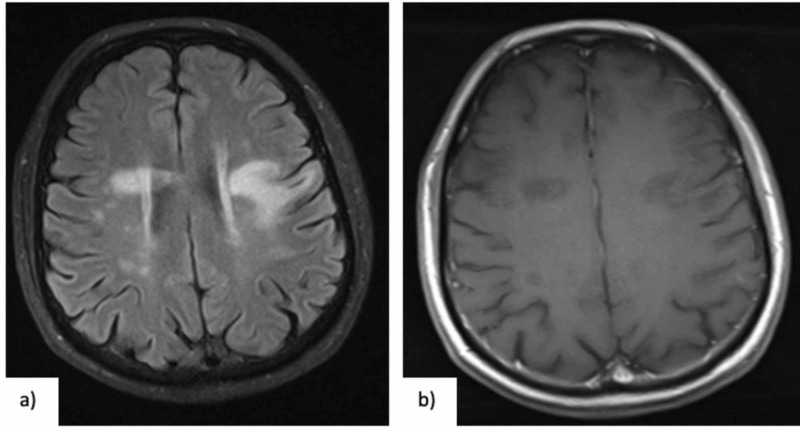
(a) Brain MRI (05-07-2017) performed after corticotherapy, showing reduction of previous lesions, in T2 FLAIR (axial), and (b) correspondent reduction of hypointense lesions in T1 (axial), with almost no gadolinium enhancement.
